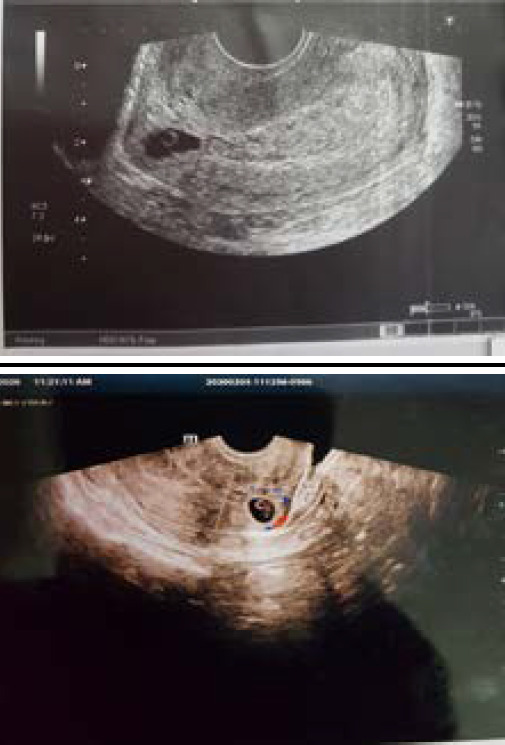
Transvaginal sonography 1st (1A) suggestive of scar pregnancy, Second Transvaginal sonography 2 days after the first Transvaginal sonography suggestive of cervical pregnancy (1B).
